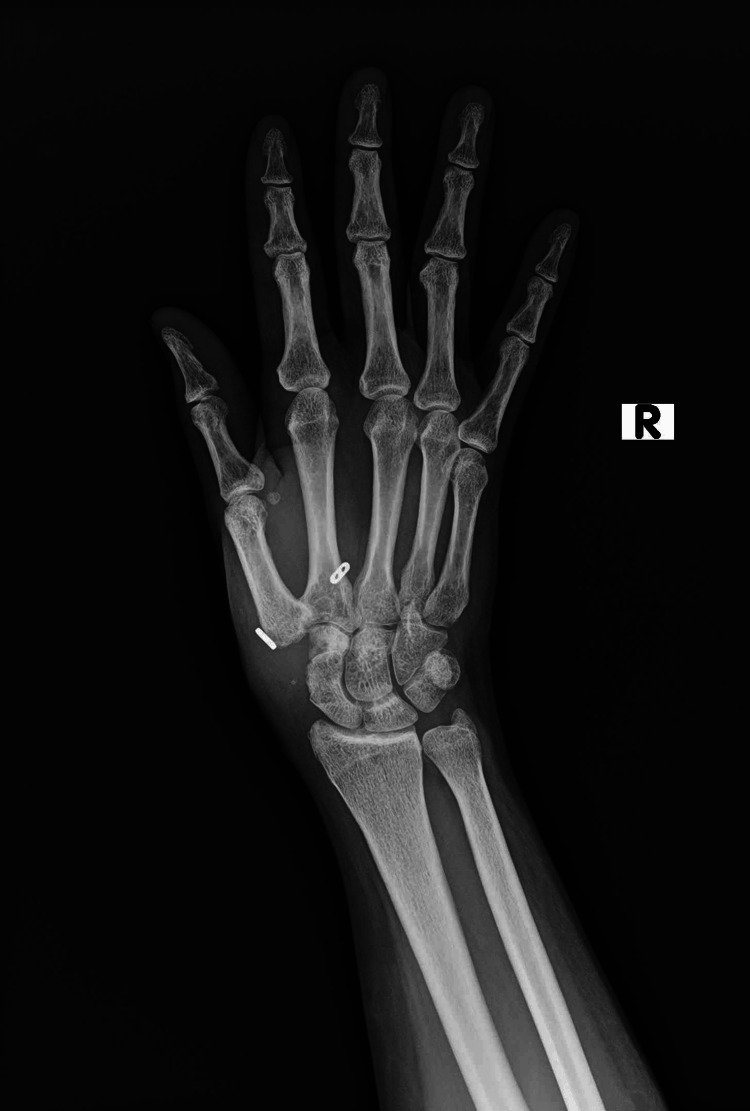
Posteroanterior radiograph of the right hand at one week post first carpometacarpal joint arthroplasty revision surgery with suture button suspensionplasty. Maintenance of the metacarpal suspension with a proper alignment of the first metacarpal can be seen.
